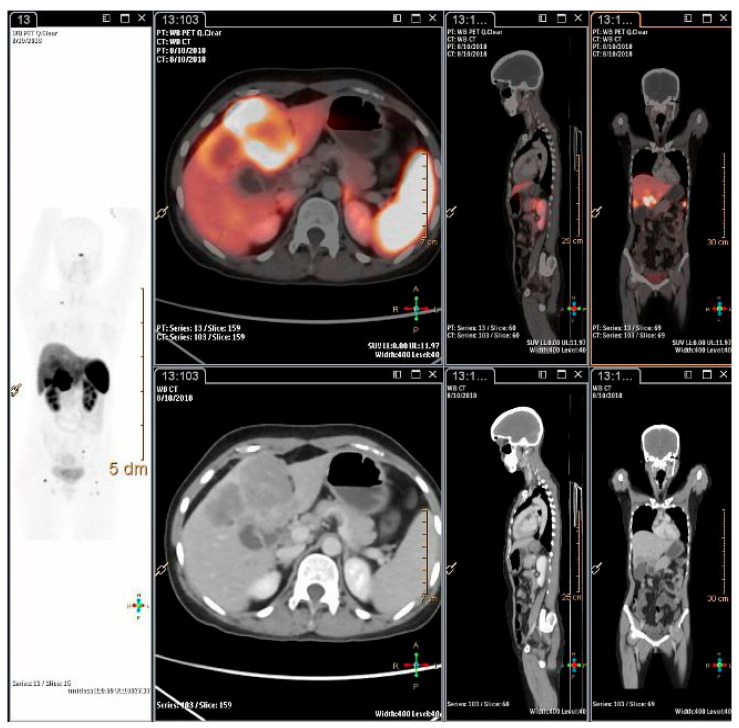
A 26-years-old lady, presented with pain abdomen and was evaluated for the same. CECT abdomen showed multiple liver lesions and abdominal lymph nodes. Biopsy and IHC from liver lesion showed high grade neuroendocrine carcinoma, large cell variant (Ki-67 index 80%). She was referred for opinion for PRRT. ^68^Ga-DOTATATE PET-CT scan showed intensely SSTR expressing polypoidal gall bladder mass infiltrating into inferior surface of liver with SSTR expressing multiple liver lesions, abdomino-pelvic lymph nodes and SSTR expressing lesion in head of right femur.
